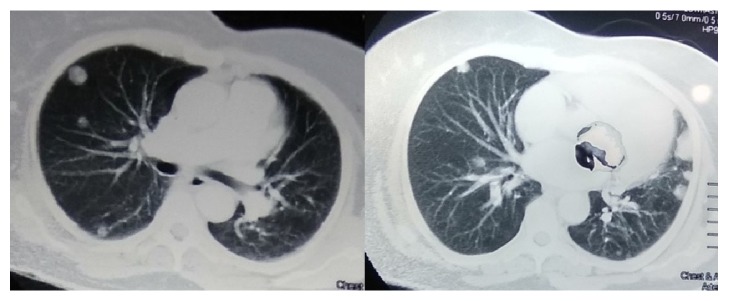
Bilateral pulmonary metastasis in middle and lower zones in CT of chest.
